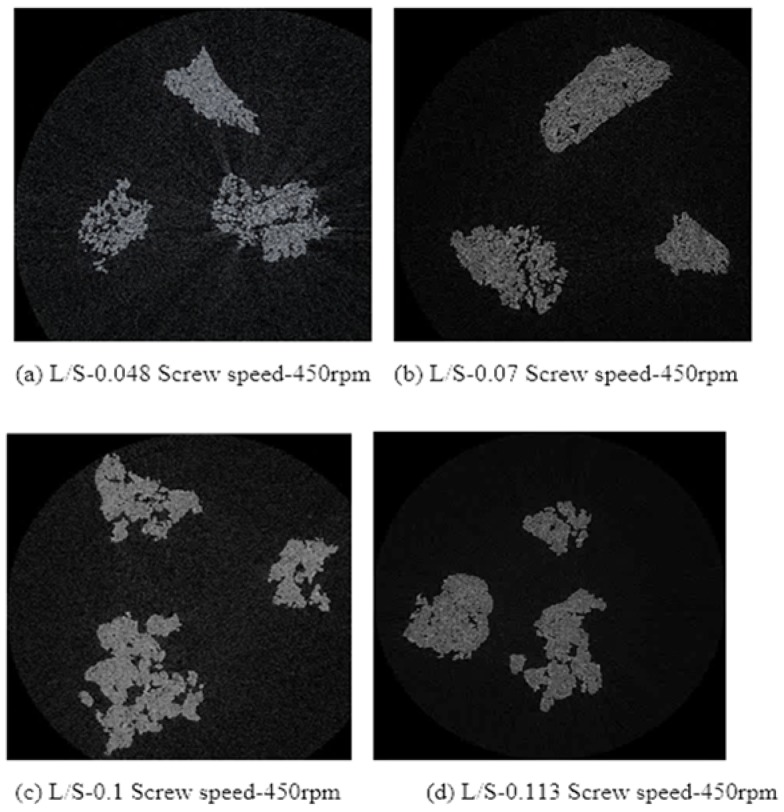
X-ray tomographic images of granules produced using SuperTab11SD at varying L/S (screw speed, 450 rpm).
